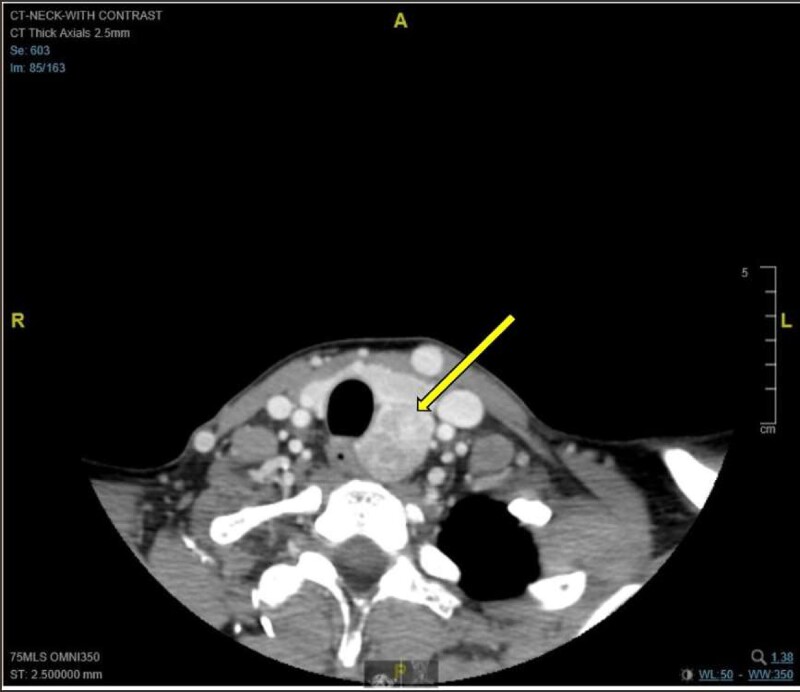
Axial CT image with contrast demonstrating a moderately avid heterogeneously enhancing exophytic mass at the posterior aspect of the left thyroid lobe, which measures 5.2 cm in the craniocaudal dimension, 2.3 cm in the mediolateral dimension, and 2.6 cm in the anteroposterior dimension. The mass extends to the left posterior paraesophageal location with mild deviation of the trachea and the esophagus to the right. There is no invasion of the surrounding structures. The remainder of the thyroid gland is normal.
